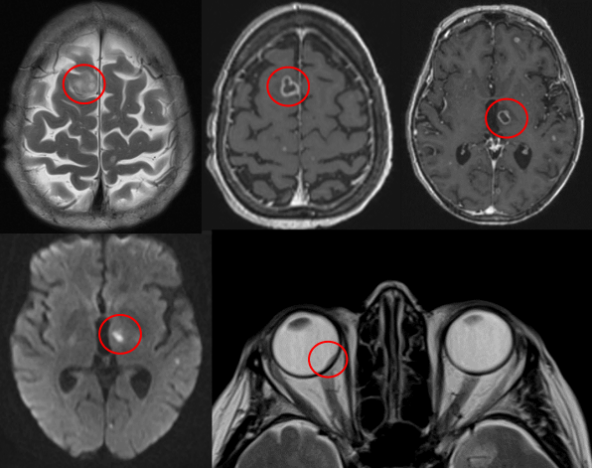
MRI brain showing disseminated cerebral nocardiosis and choroidal abscess
